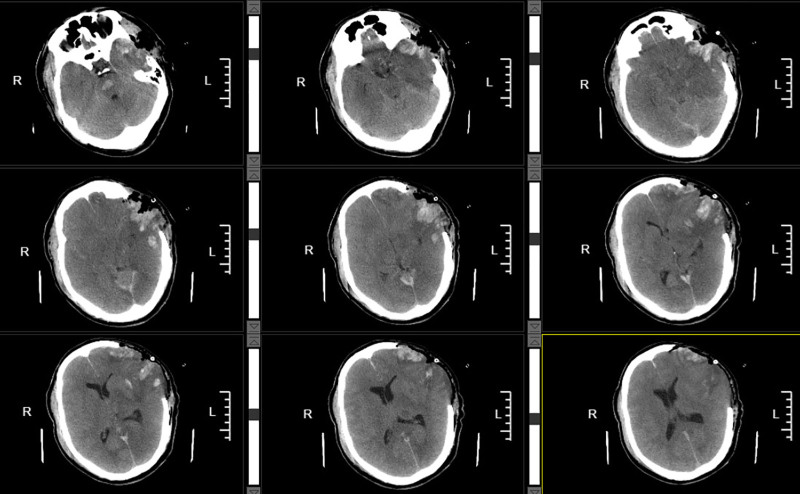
CT scan demonstrated restoration of midline structures, preservation of the ambient cisterns, and evidence of hemorrhage within the decompressed brainstem. CT = computed tomography.
